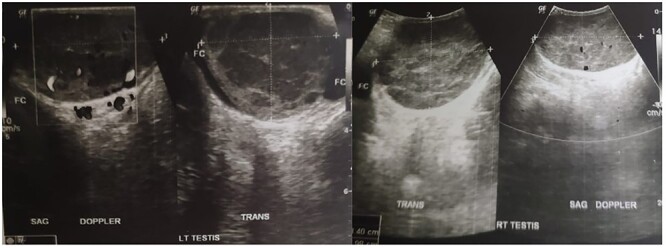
Testicular ultrasonography result.
